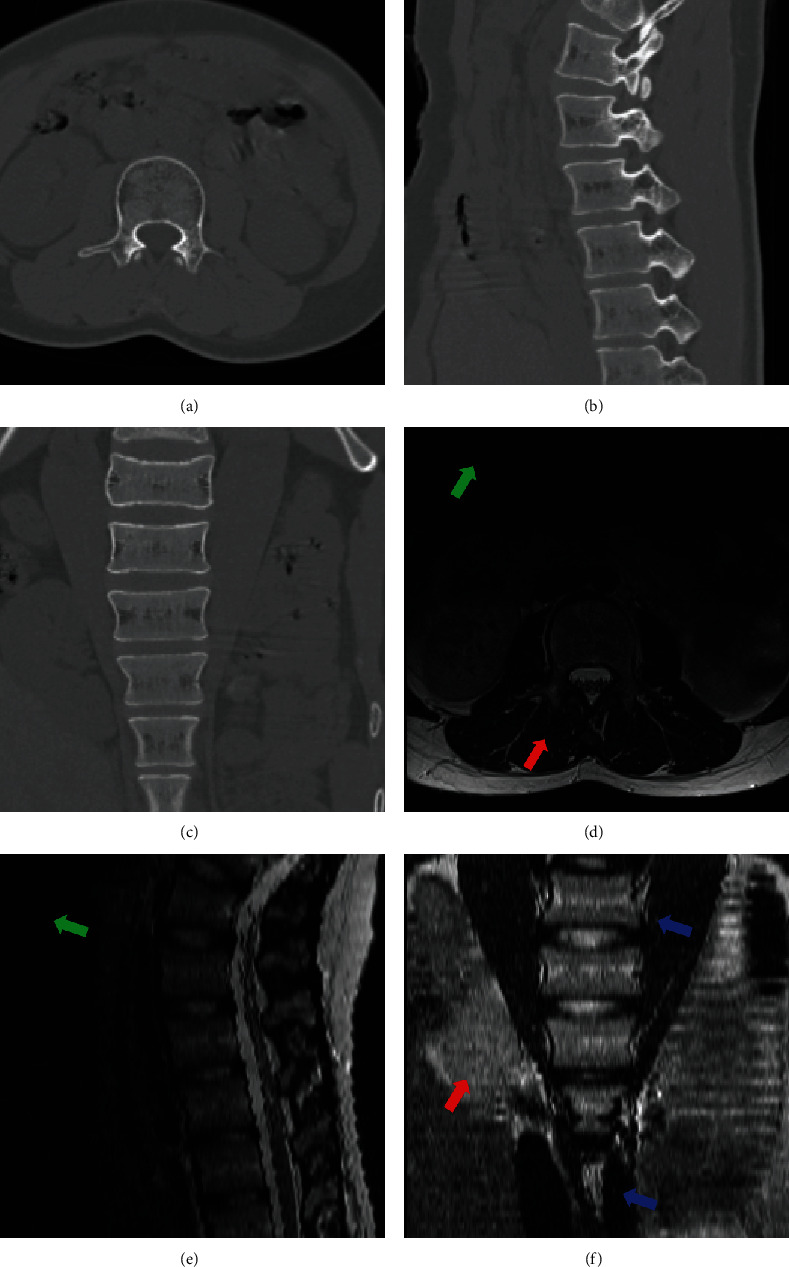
Perceived visual difference between CT and MR images of lumbar spine from three perspective views. The difference of imaging characteristics, fields of view, and unavoidable motion make the registration challenging. Red arrows show different imaging contrast, green arrows direct to the undesirable artifact of bias field in MR images, and blue arrows indicate different field of views. Note that images are cropped and scaled for display purpose.
